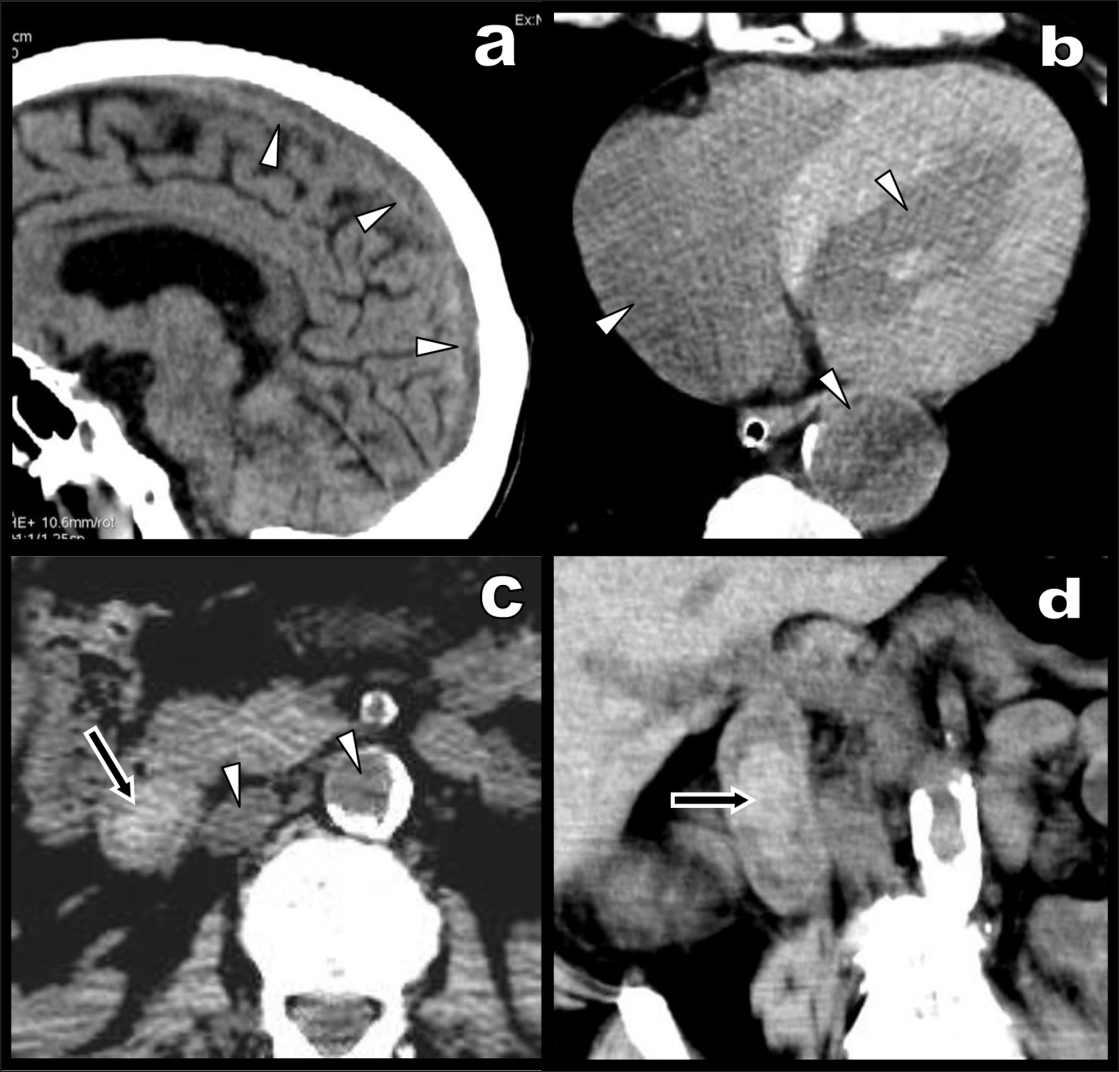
Unenhanced CT views: (a) Sagital MPR view obtained at the level of the longitudinal venous sinus of the brain shows hypodensity of blood (white arrowheads); (b) marked hypodensity of blood (25–30 UH) is also found in the cardiac cavities (white arrowheads); axial (c) and coronal MPR views (d) reveal a spontaneously dense fresh clot in the 2^nd^ duodenum (black arrow). Its high density (60 UH) contrasts with the high hypodensity of blood (25–30 UH) in the great abdominal vessels (white arrowheads).
